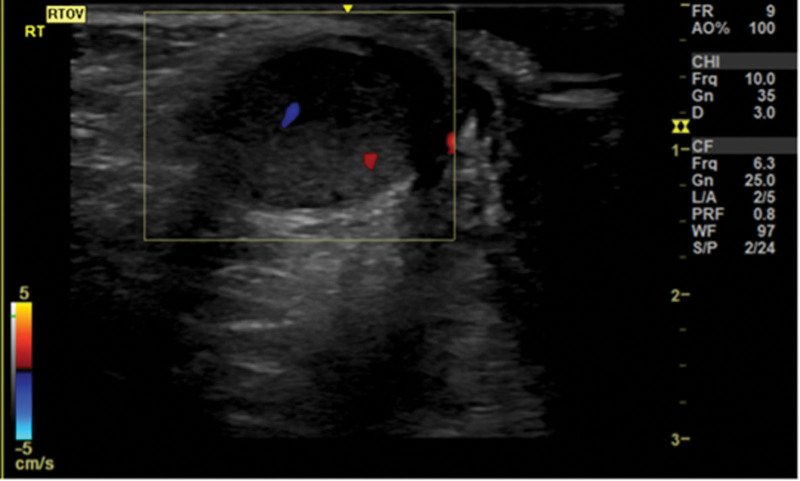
Doppler ultrasound of the right testicle with preserved of flow and homogenous content.
